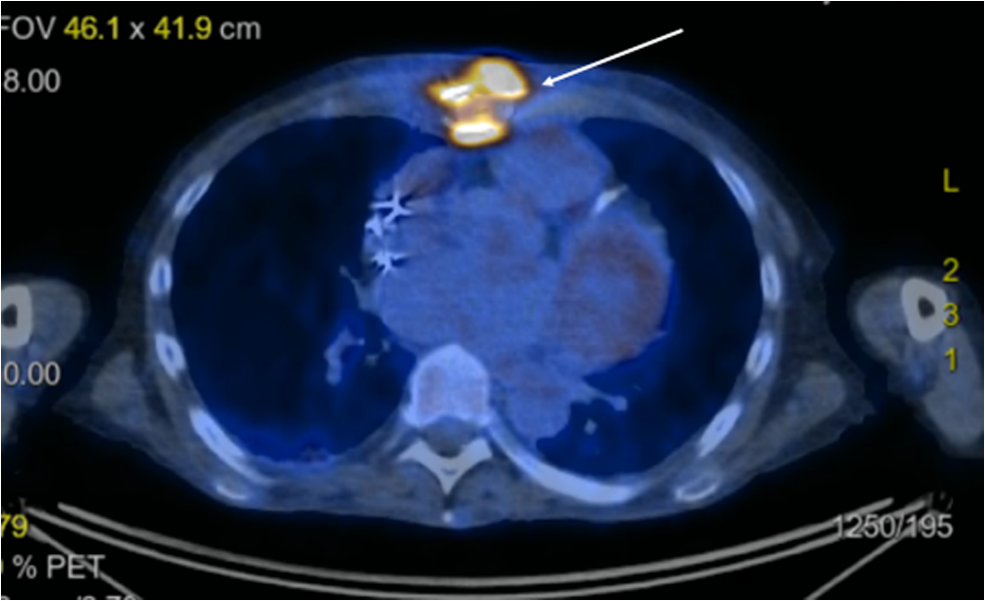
Sternal uptake in a fluorodeoxyglucose positron emission tomography (FDG-PET) scan
